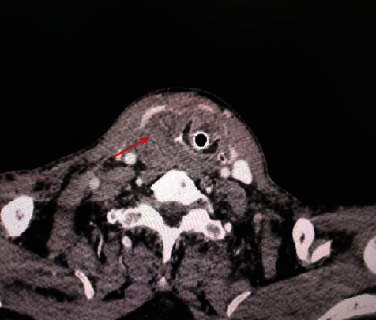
The CT of the neck in patient 4, showing occupation of the right thyroid lobe.
